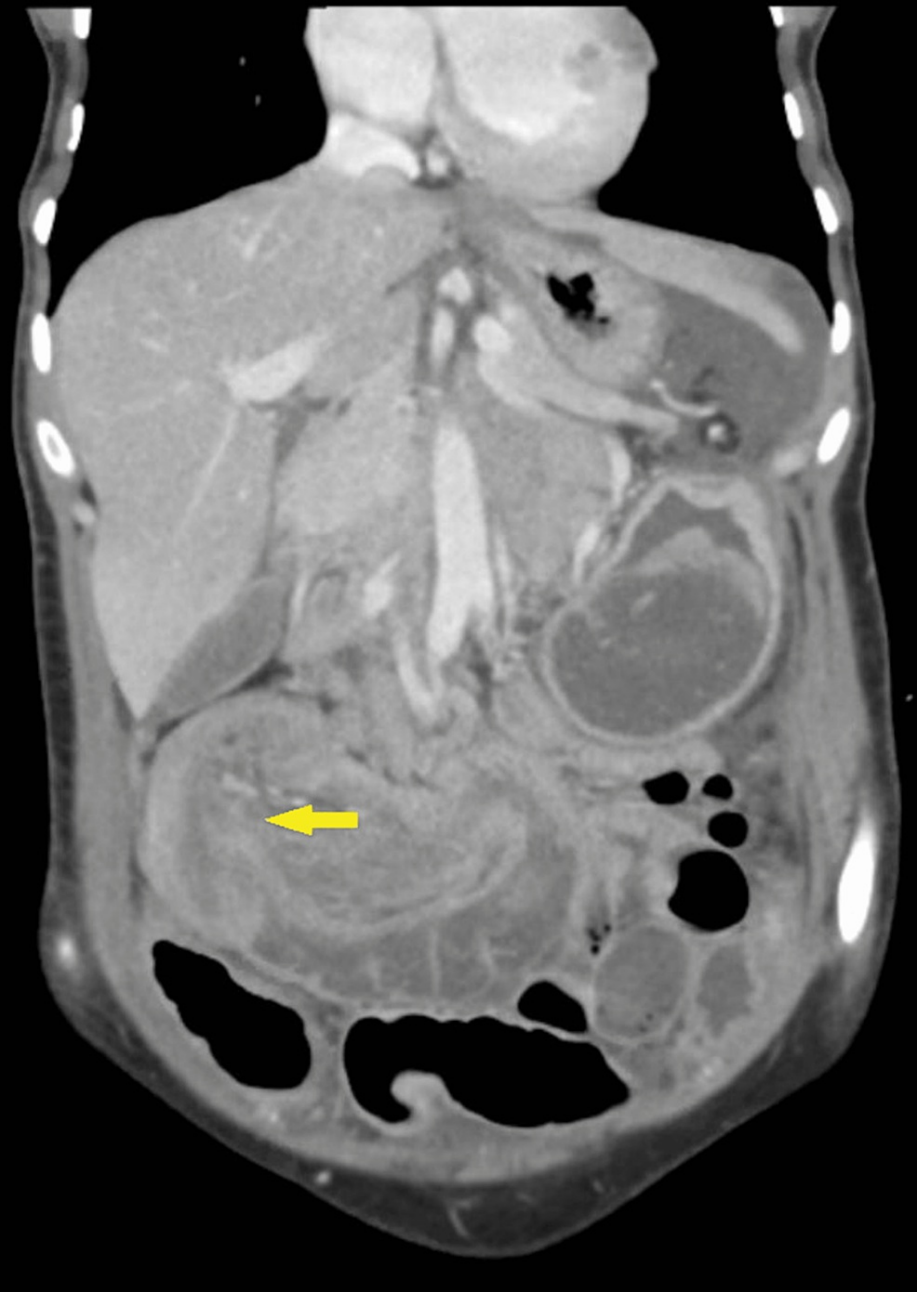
Cecal-colonic intussusception in the coronal cut of abdominal computed tomography scan (yellow arrow)
